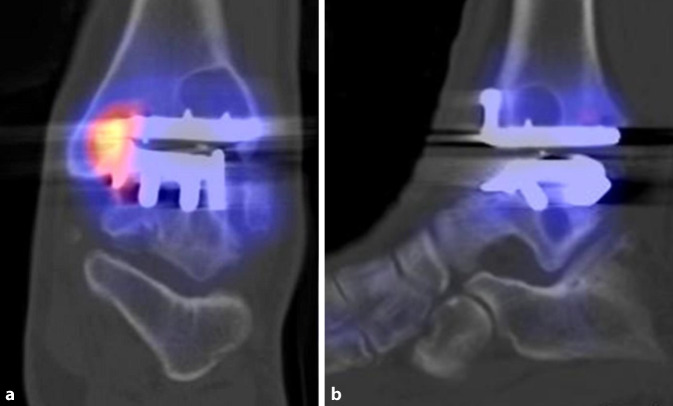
## SPECT/CT beim diabetischen Fuß
